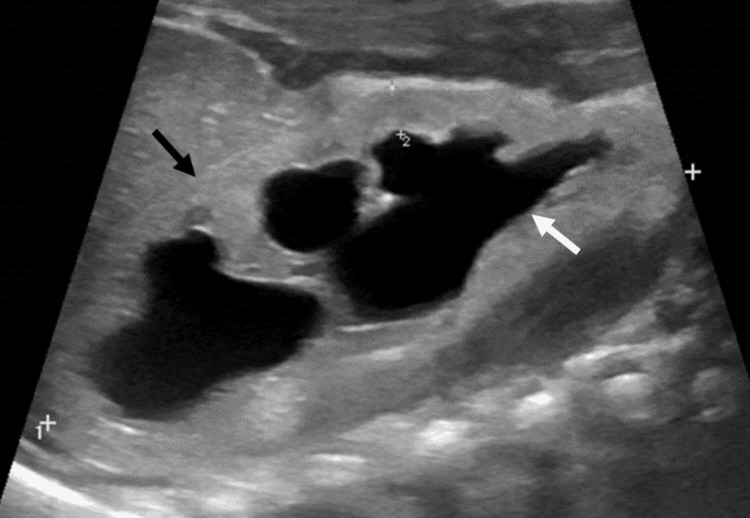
Ultrasound showing dilation of central and peripheral calyces (white arrow) with thinning and dysplastic changes of the renal parenchyma (black arrow).
